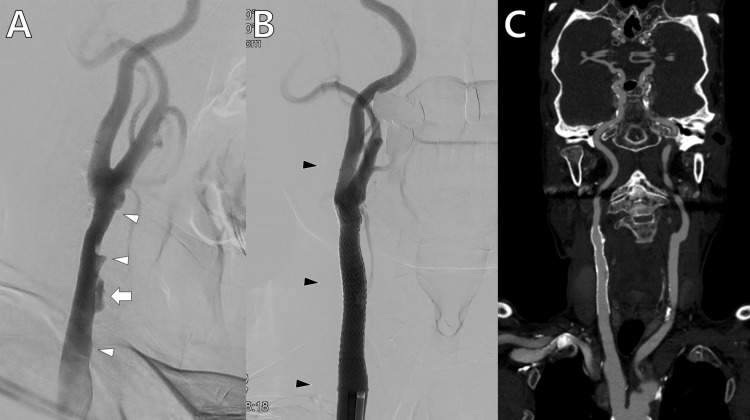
Intra- and post-operative radiological findings (A) Carotid angiogram showed an irregular intimal flap (white arrowheads) with the false lumen (white arrow) and the remaining mural hematoma. (B) Stenting (black arrowheads) is performed using two stents in a telescoping configuration with an overlapping at the mid portion. Note that the false lumen is completely collapsed and the true lumen is fully enlarged. (C) Coronal section of the head-neck computed tomography angiography a week later shows a patent flow within the carotid stents.
